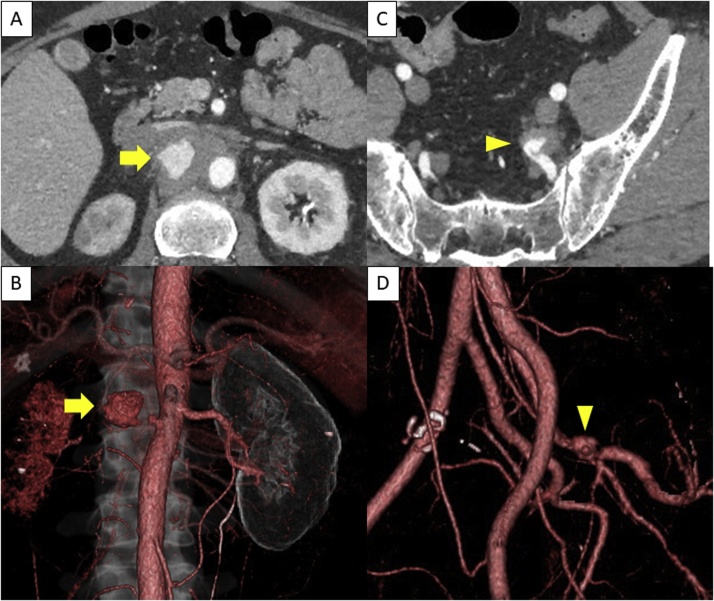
Preoperative computed tomography. (A) Blood leaked from the vessel and pooled at the right of the aorta (arrow) and (B) the right renal artery is not enhanced. The left internal iliac pseudoaneurysm (C) was also observed as a saccular aneurysm (D) (arrowhead).
